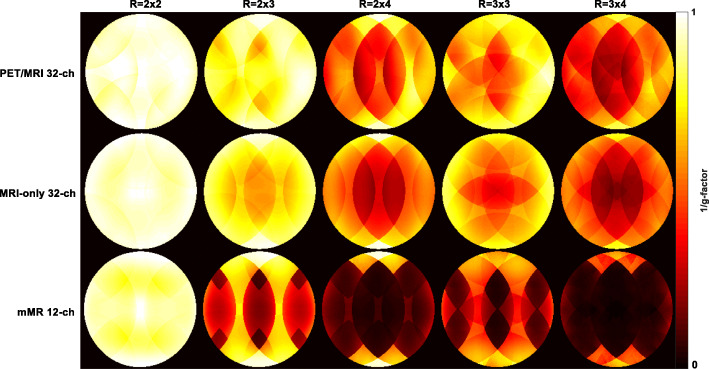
1/g factor maps in the case of 2D acceleration with *RR* = 2 × 2 to *RR* = 3 × 4. The PET/MRI 32-channel array achieves the least noise amplification with max of 0.97 at *RR* = 3 × 3 in comparison to 0.88 for the MRI-only array, while the mMR 12-channel array produced the maximum noise amplification of 0.83
